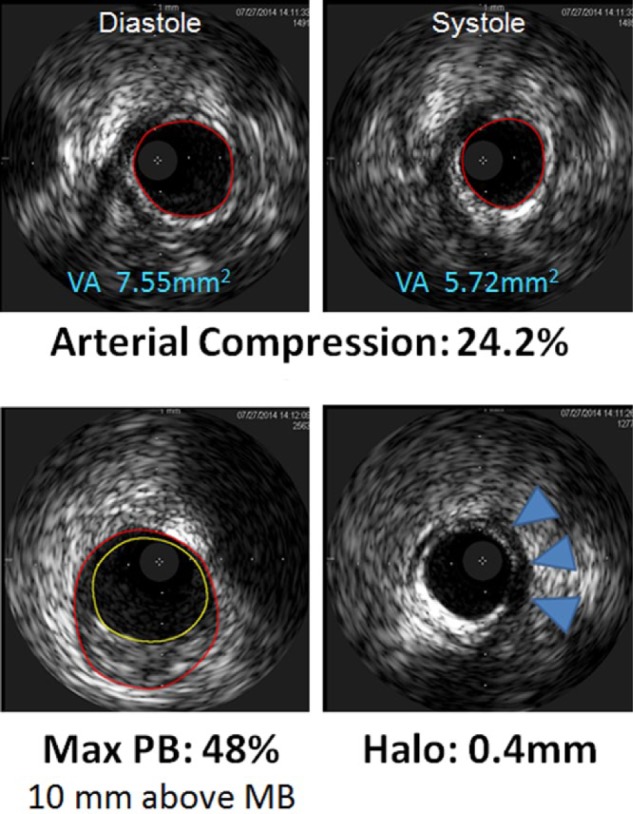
Top panel: IVUS still frames demonstrating cross-sectional images of vessel area from within the MB resulting in an arterial compression of 24.2%. Bottom panel: The maximum plaque burden (PB) was 48%. The halo (MB thickness), blue arrows, was 0.4 mm. MB, myocardial bridge; VA, vessel area; IVUS, intravascular ultrasound.
